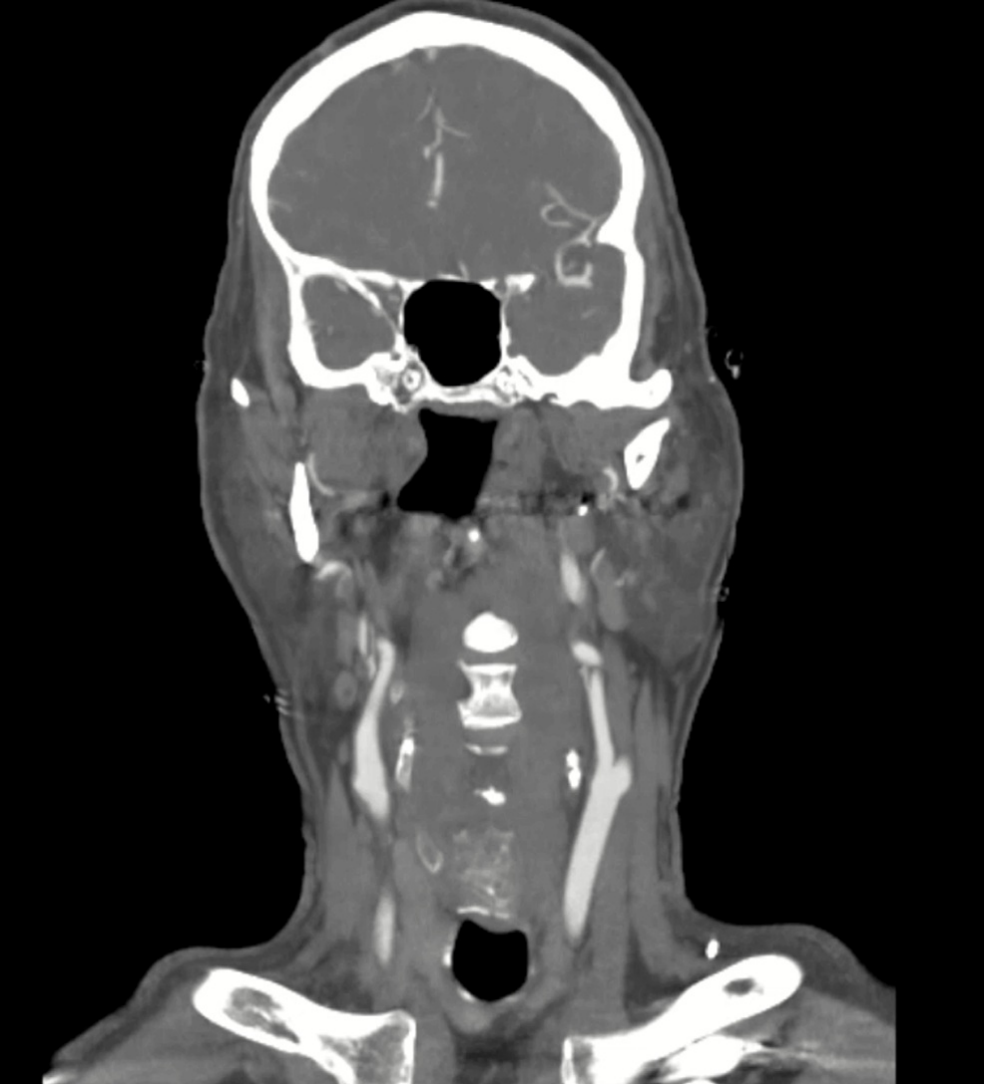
CT angiography of the head and neck revealing no significant large vessel occlusion or stenosis, or perfusion abnormalities CT: computed tomography
